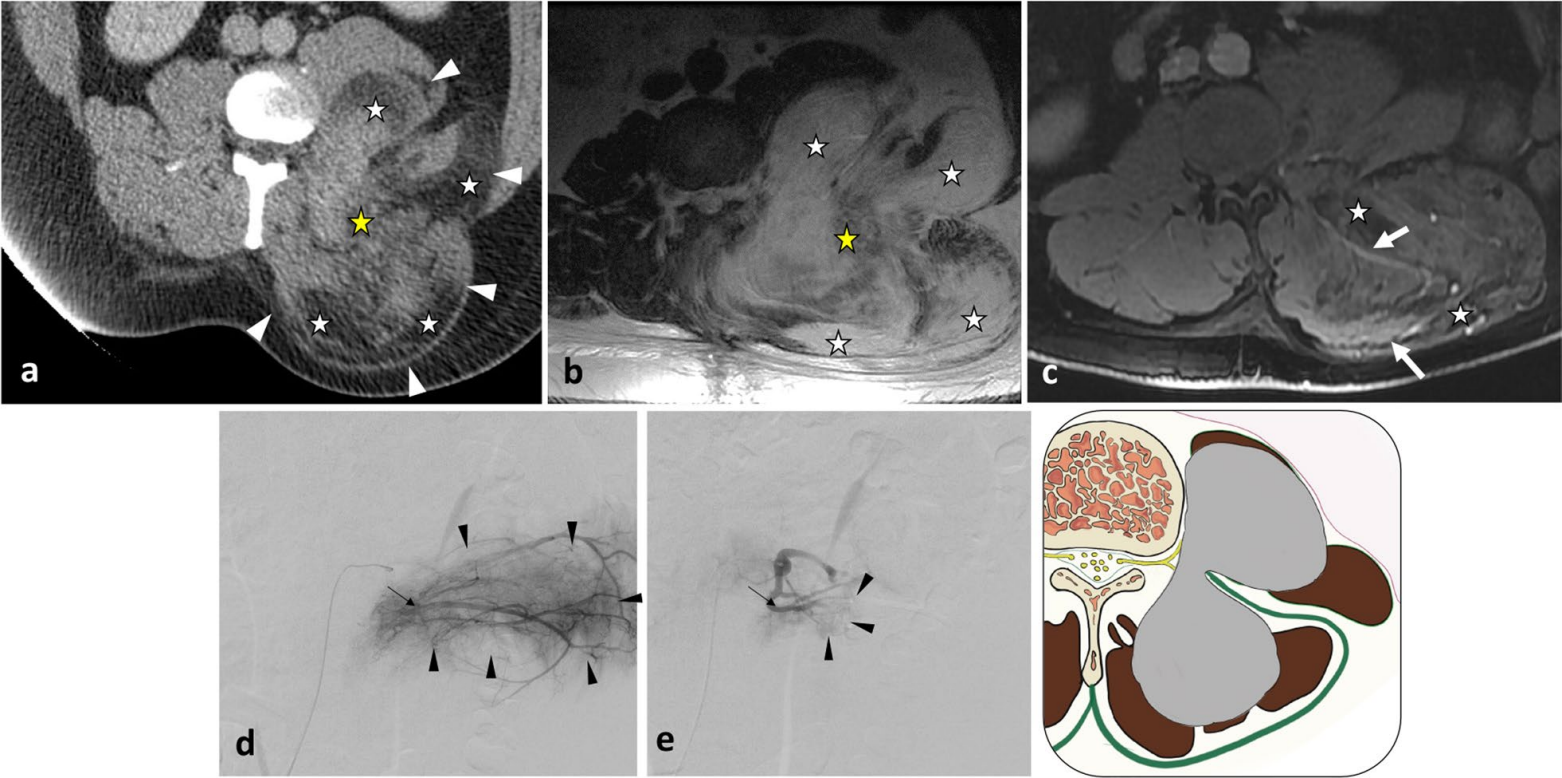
Liposarcoma* of the left lumbar paraspinal muscles in a 57-year-old male with a bump in the back and mild left iliac fossa pain. Contrast-enhanced axial CT scan reconstruction (a) and axial T1WI (b) show intramuscular lipomatous tumour invading both the hypaxial and epaxial muscles. Fat content (white stars) presents low attenuation on CT, high signal on T1WI and saturates on fat-saturated T1WI (c). The mass demonstrates a bilobed shape with an epicentre located between the epaxial and hypaxial regions (yellow stars). Axial contrast-enhanced fat-saturated T1WI (c) shows muscle and fascia enhancement (arrows), a key driving feature for liposarcoma. Digital subtraction angiogram (d) of the left 2^nd^ lumbar artery (arrow) shows hypervascularisation of the tumour (arrowheads). After embolisation (e), hypervascularisation decreased (arrowheads)
